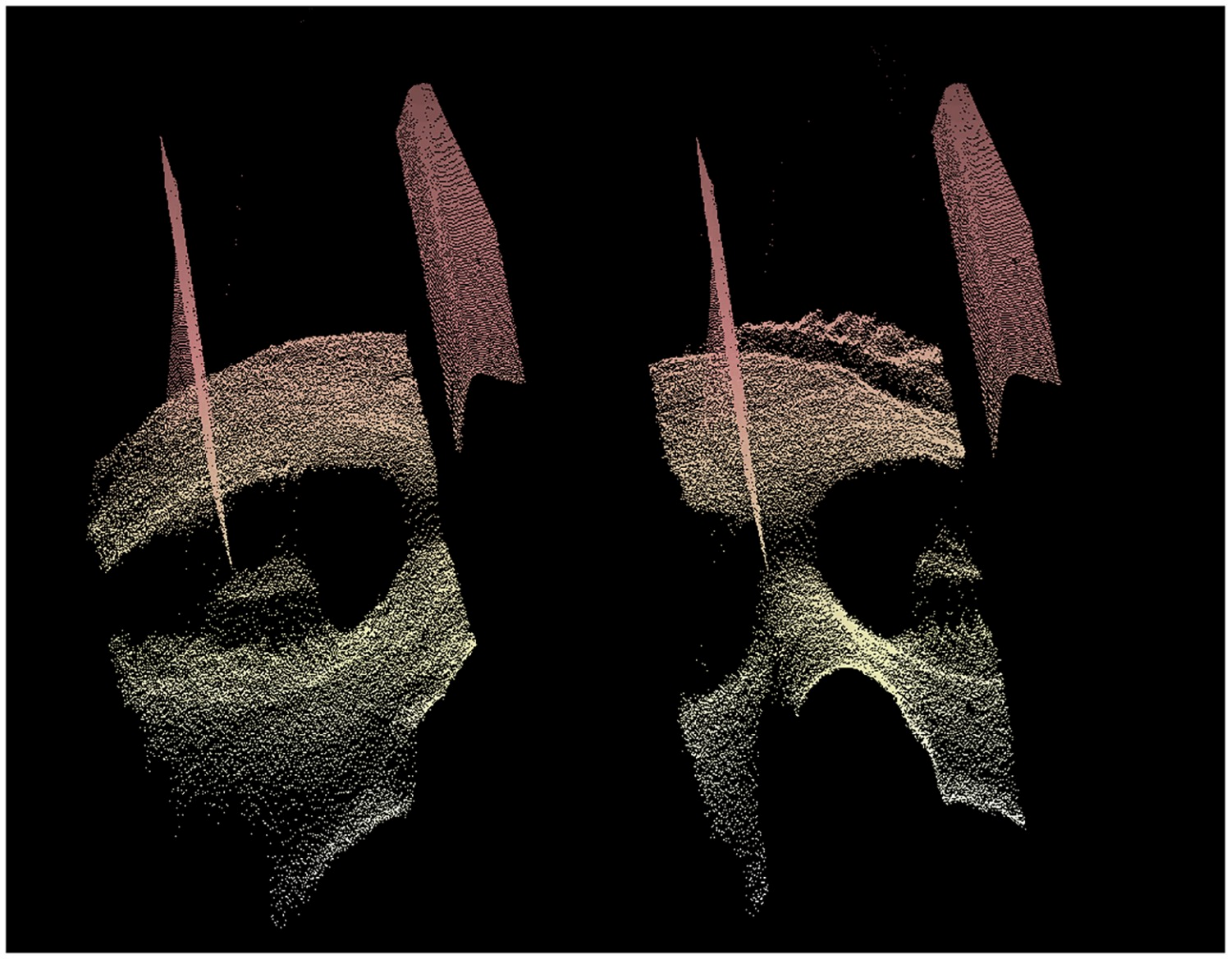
3D facial point clouds of a subject. 3D facial point clouds of a subject inside an MRI head coil obtained during MRI acquisition using the TCL2 for motion tracking. The two point clouds are obtained at different times during the acquisition. Part of the MRI head coil is seen in front of the facial surface of the patient. It is clearly visible, that the head of the subject has moved relative to the head coil during the acquisition.
